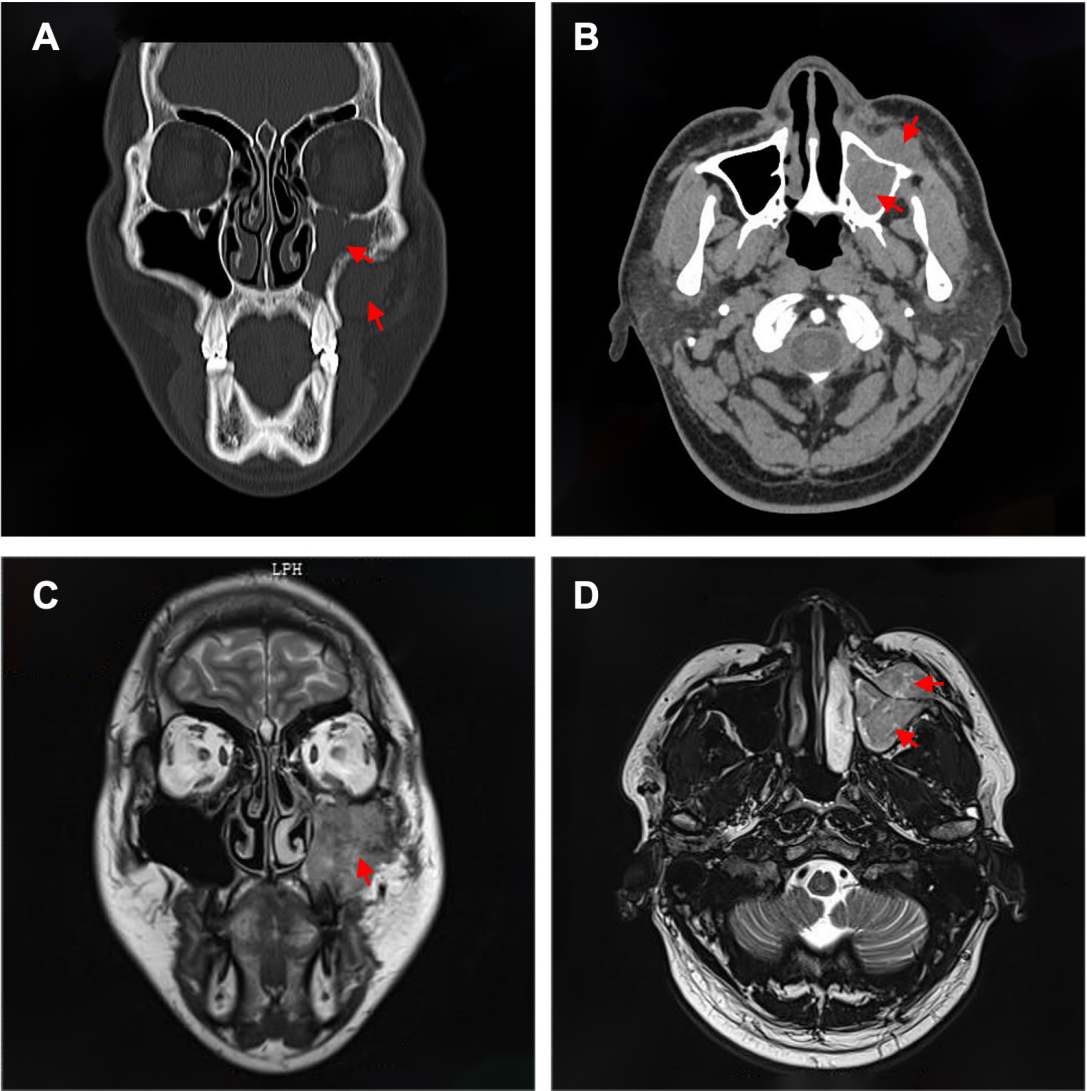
Preoperative imaging of this case. (A) Axial and (B) coronal CT images showing a soft-tissue mass occupying the left maxillary sinus with erosion and thinning of the anterior and inferior sinus walls. (C) Axial and (D) coronal contrast-enhanced MRI images demonstrating heterogeneous enhancement of the lesion with extension into the maxillofacial soft tissue.
